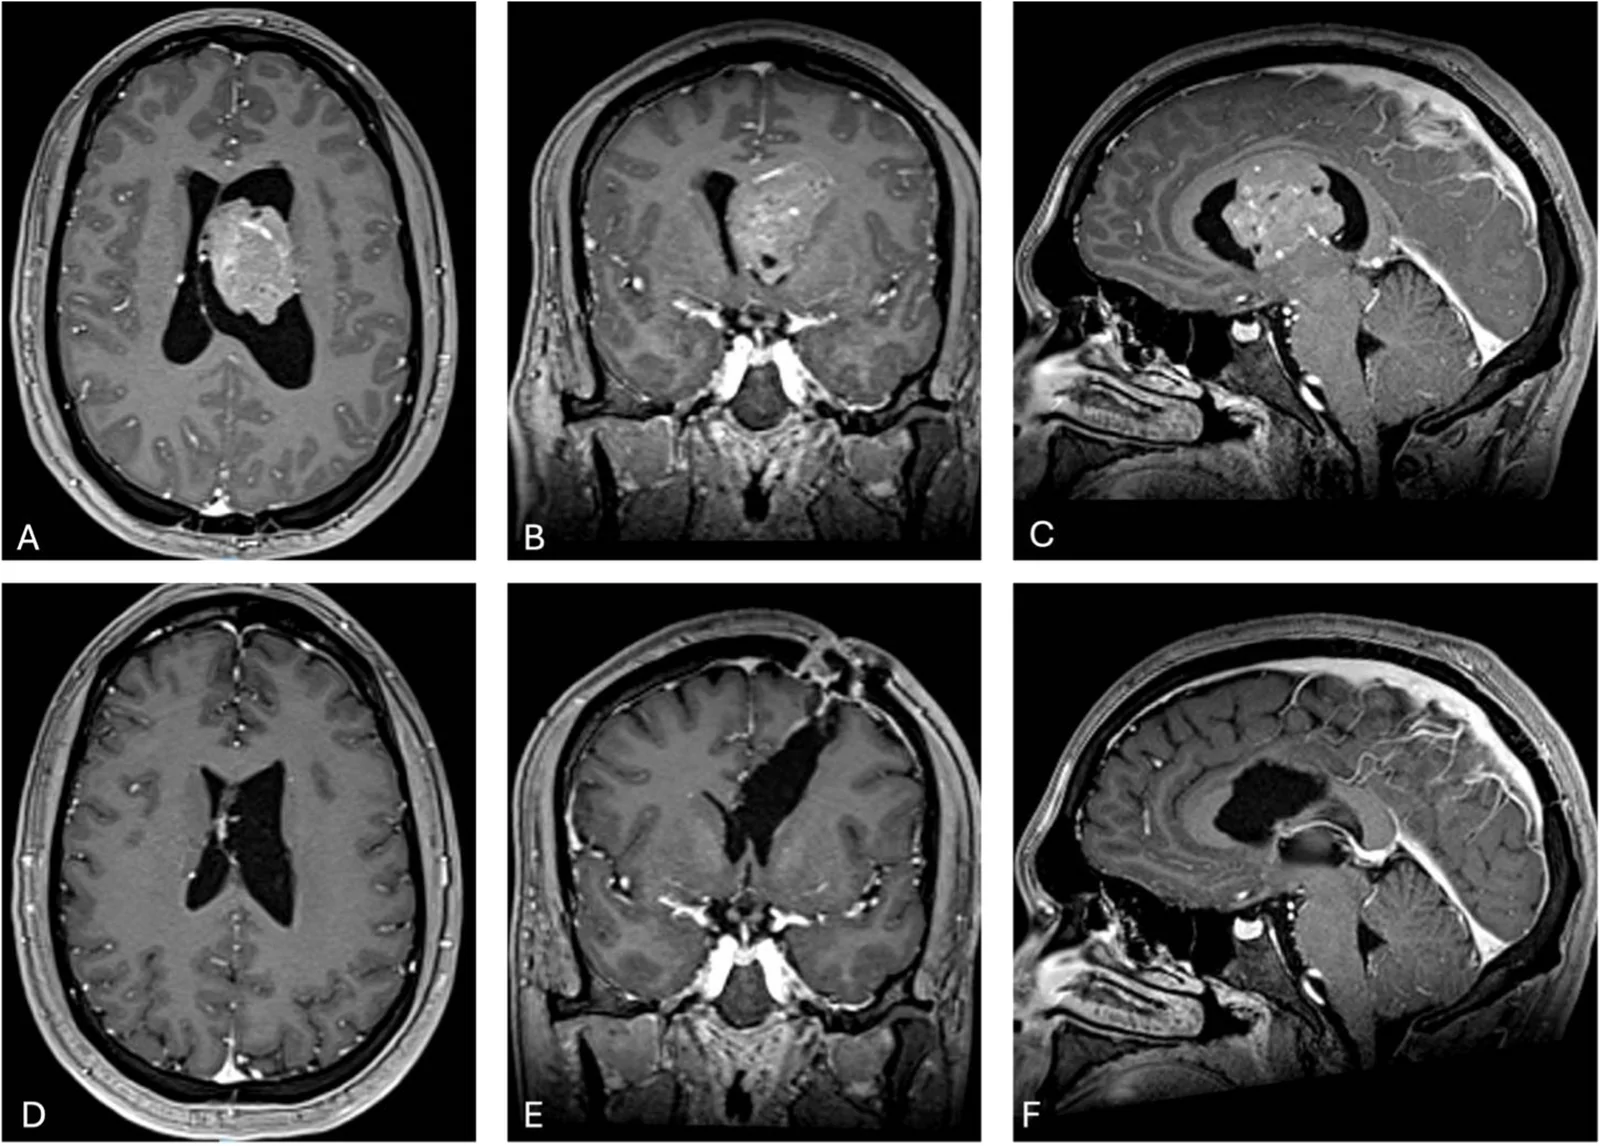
Preoperative brain MRI, axial (A), coronal (B) and sagittal (C) sequences. Solid and highly vascularized lesion, with regular margins, heterogeneous signal intensity and mild, non-uniform enhancement, was evident in the left ventricle. Intralesional microvascular flow voids were also observed. Postoperative brain MRI at last follow-up, axial (A), coronal (B) and sagittal (C) sequences. Gross-total lesion removal and no regrowth were evident
